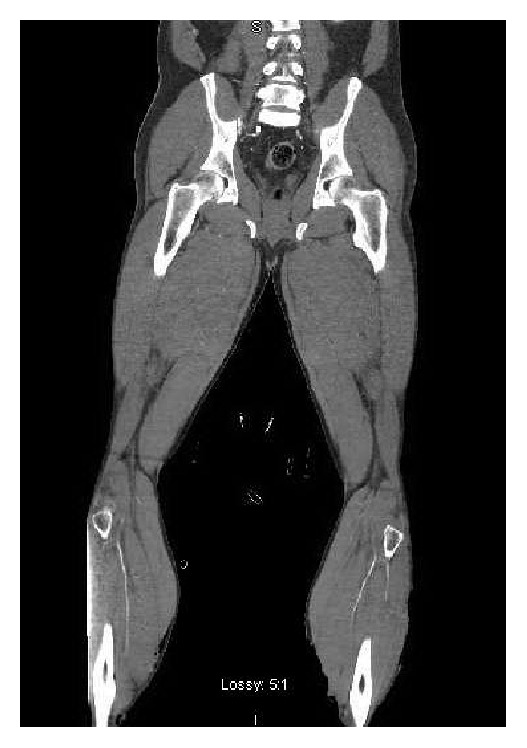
CT angiogram of the lower limbs showing marked narrowing of the distal vessels (arterial and venous) but no occlusive pathology.
